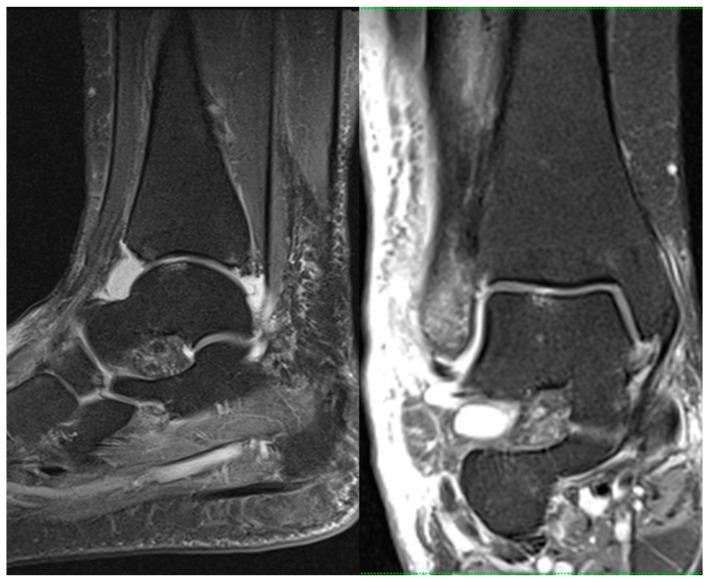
Sagittal (left) and coronal (right) proton-density-weighted turbo spin echo fat saturation MRI sequences (PD tse fs) showing CL of the talar dome in a 52-year-old female with an acute ankle fracture.
